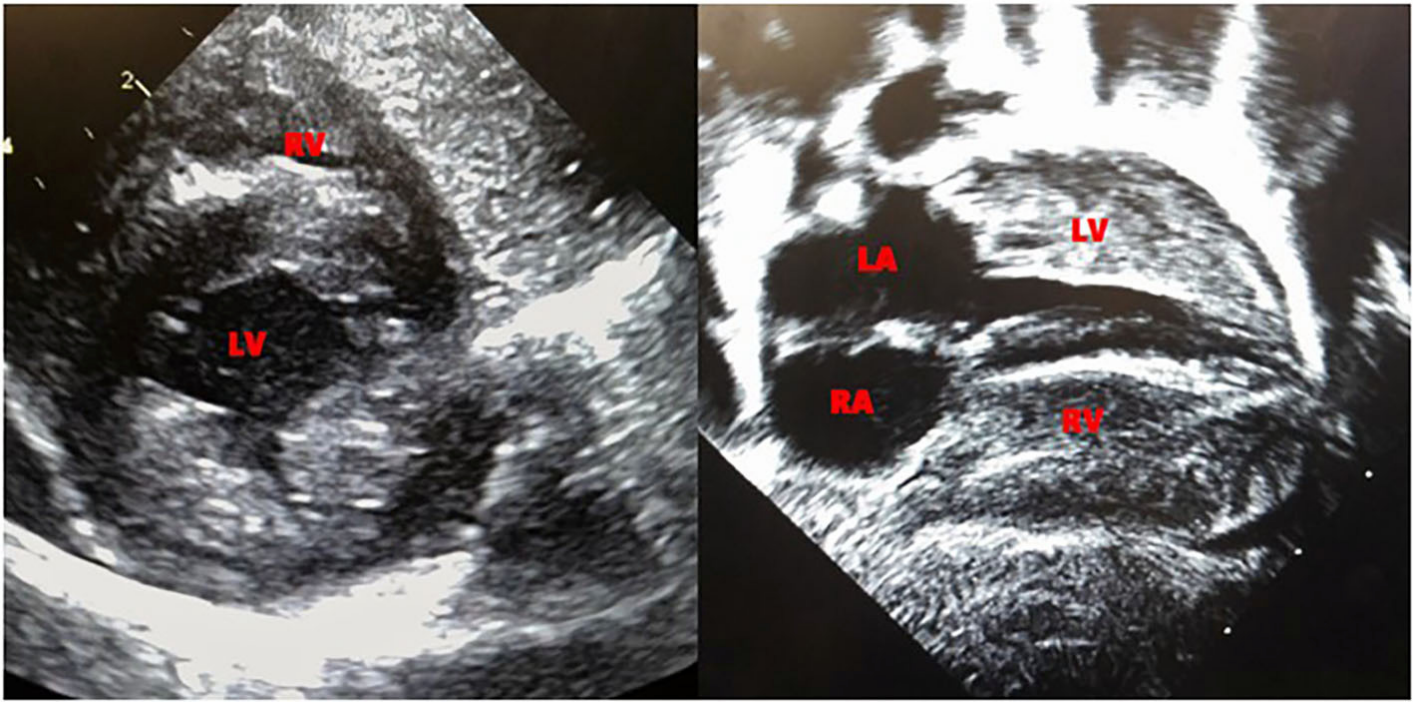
Echocardiogram at 6 days of life, showing significant biventricular hypertrophy.
